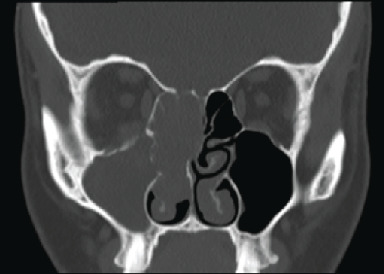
Contrast-enhanced CT sinuses (coronal section). Shows right-sided lesion within the nasal cavity associated with bony erosion and slight deviation of the upper aspect of the nasal septum to the left. There is associated complete soft tissue opacification of the frontal sinuses, ethmoidal sinuses, and maxillary sinus. Note the close relationship with the anterior ethmoid artery.
